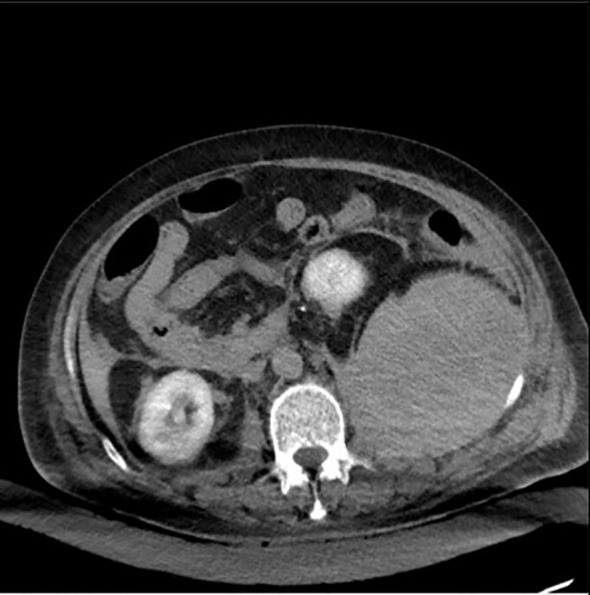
CT suggests a massive hematoma posterior to the left psoas major.
